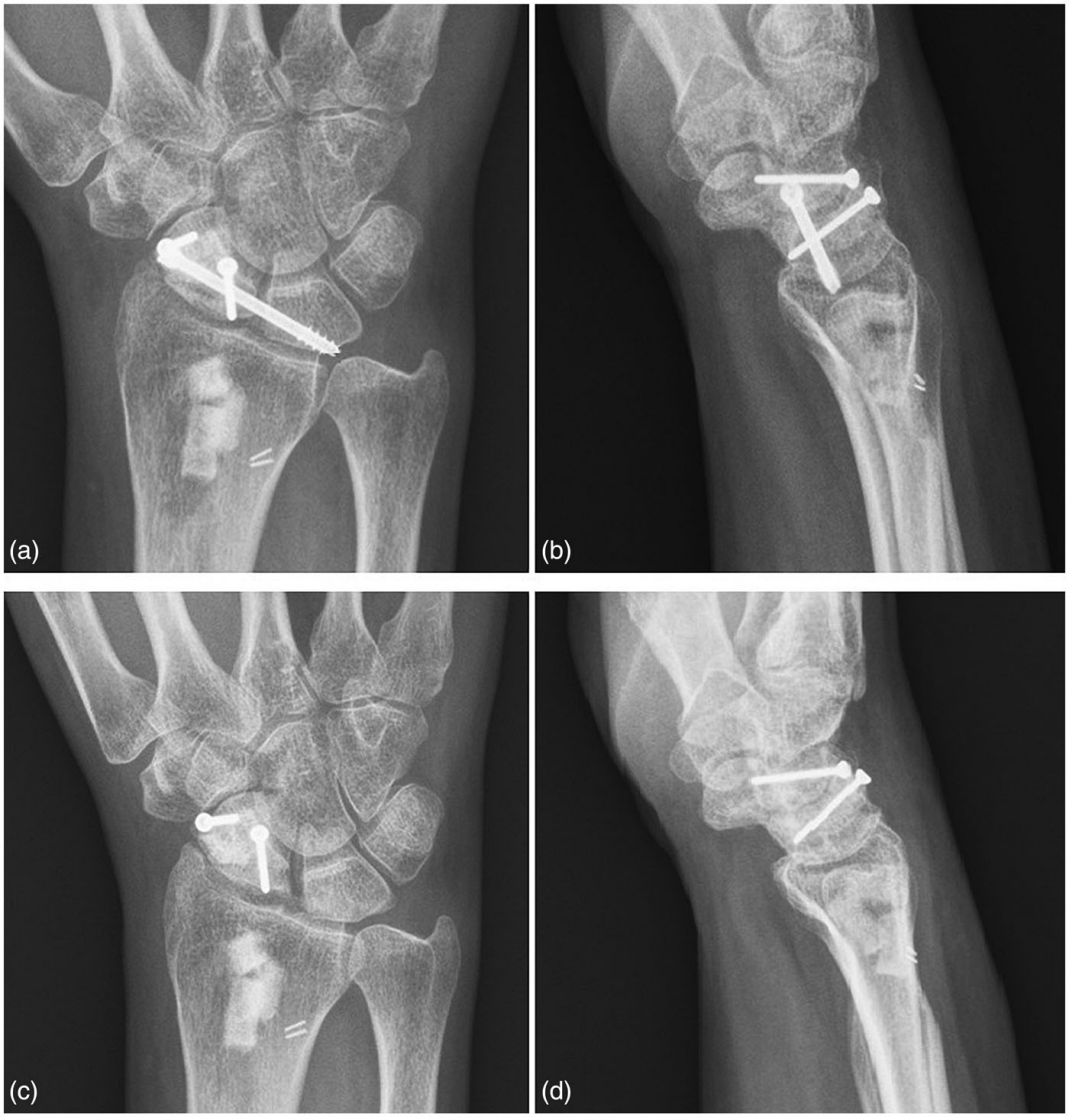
(a,b) Radiographs showing the right scaphoid after vascularized bone grafting and scapholunate screw fixation at postoperative 3 months. (c,d) Radiographs at postoperative 2 years reveal consolidated union (the screw had been removed 6 months after surgery because it was too long to penetrate the proximal cortex of the lunate).
